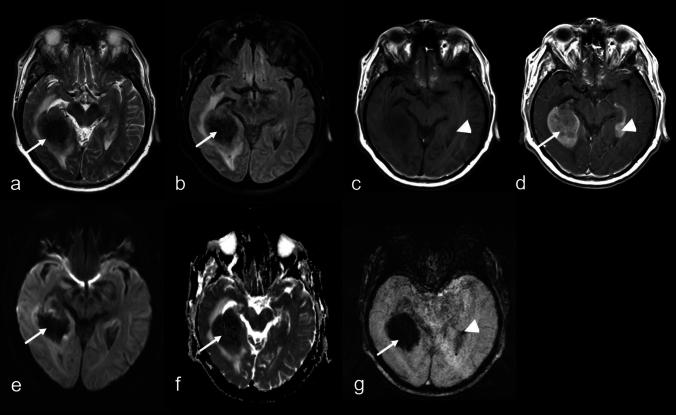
71-year-old man suffering from primary large B-cell lymphoma of immune-privileged sites (IP-LBCL), negative for Epstein-Barr virus. MRI disclosing a large solid temporooccipital right-sided lesion with conglomerate signal loss (a: T2-weighted imaging (WI); b: fluid-attenuated inversion recovery; e, f: diffusion-WI; g: susceptibility-WI; arrows.) Nearly homogeneous contrast enhancement (c, d: T1WI; arrows). Multifocal cerebral involvement is also noted, including a temporal lesion on the left side with punctate signal loss (c, d, g; arrowheads)
